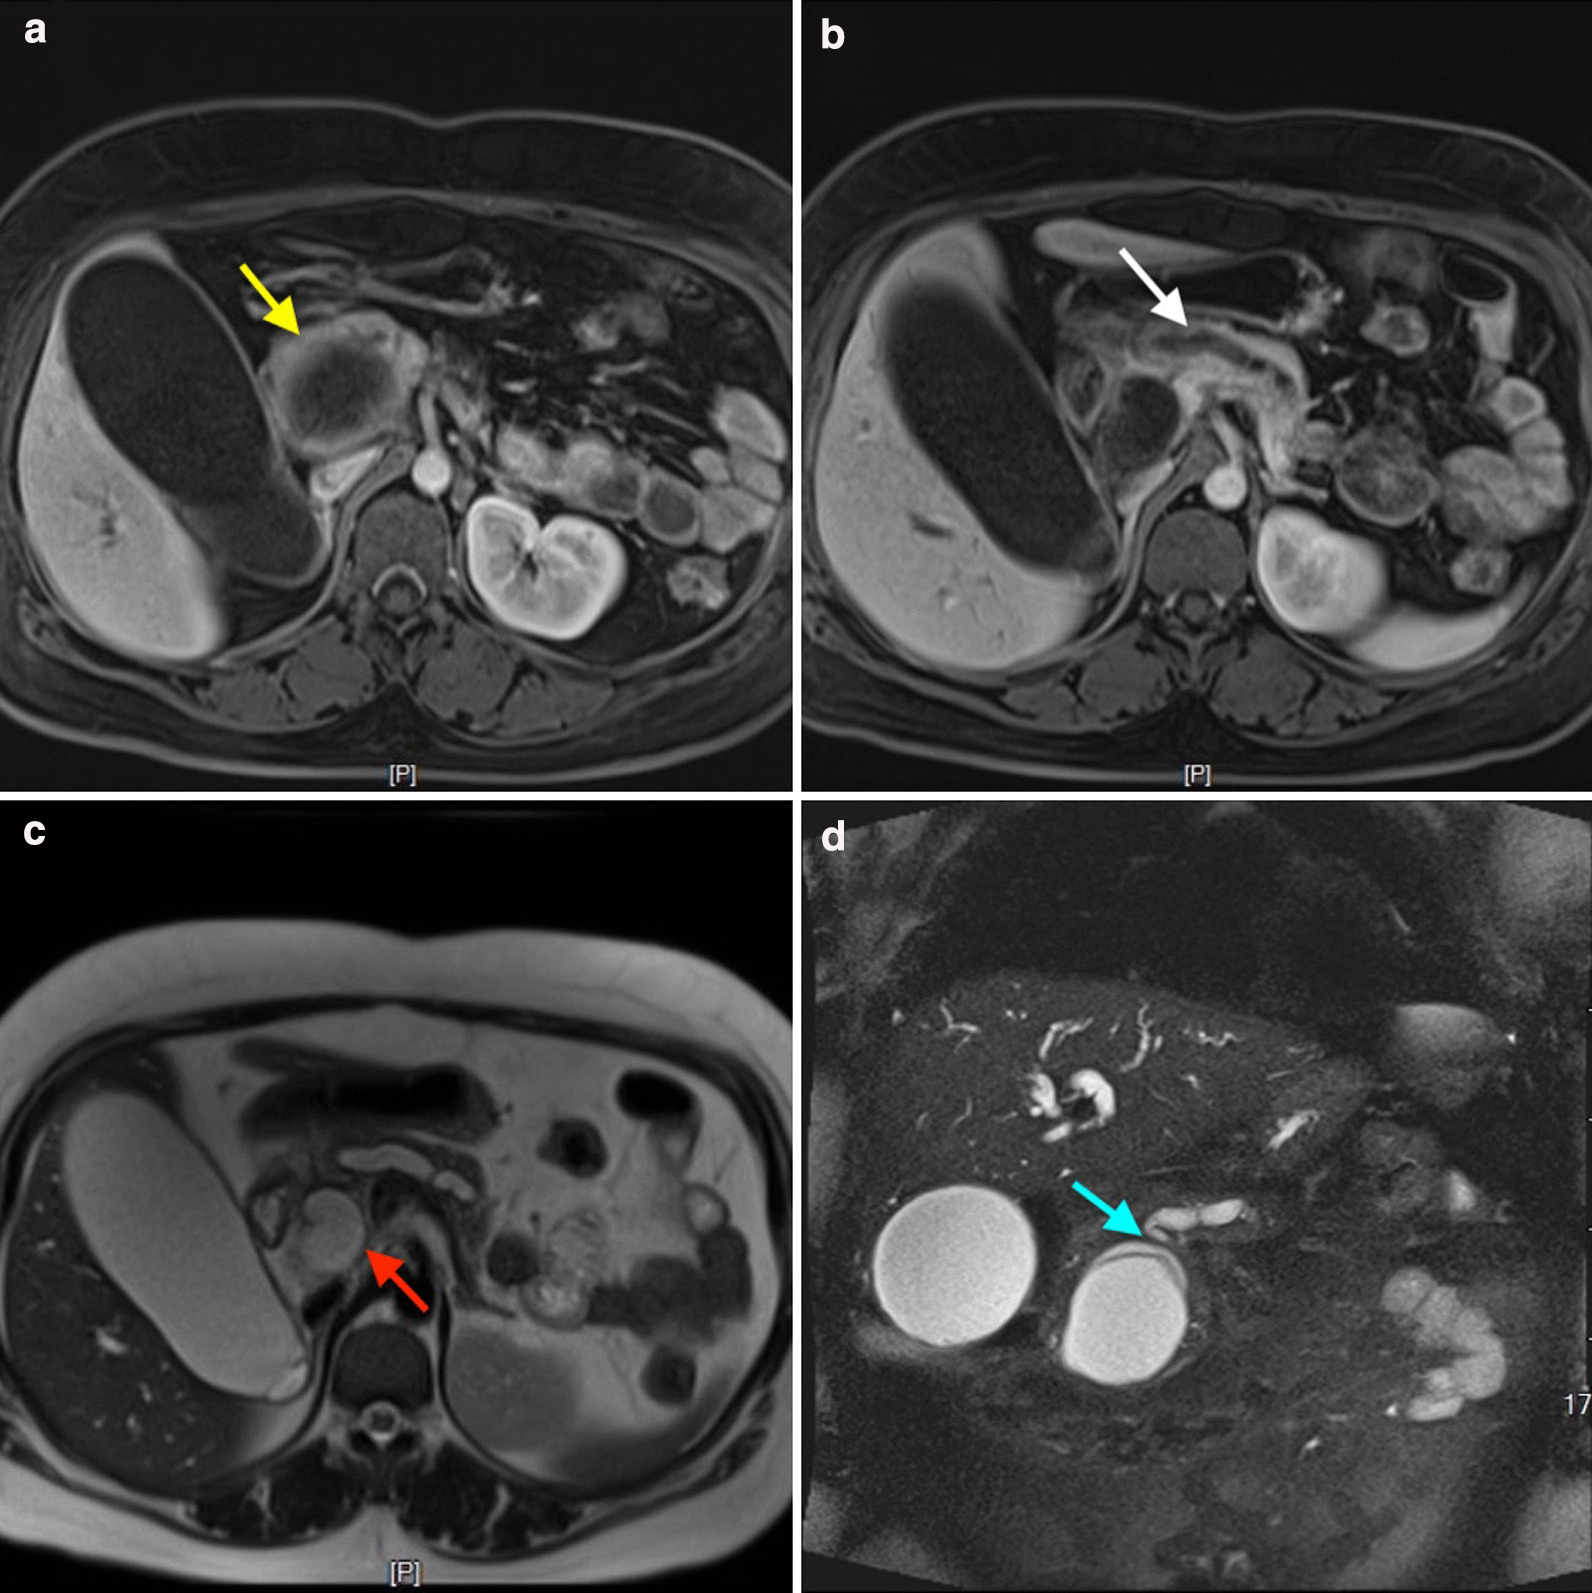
MRI of the pancreatic hydatid cyst. a The hydatid cyst mass (yellow arrow); b expanded main pancreatic duct (white arrow); c expanded bile duct (red arrow); d the hydatid cyst invaded the main pancreatic duct (blue arrow)
